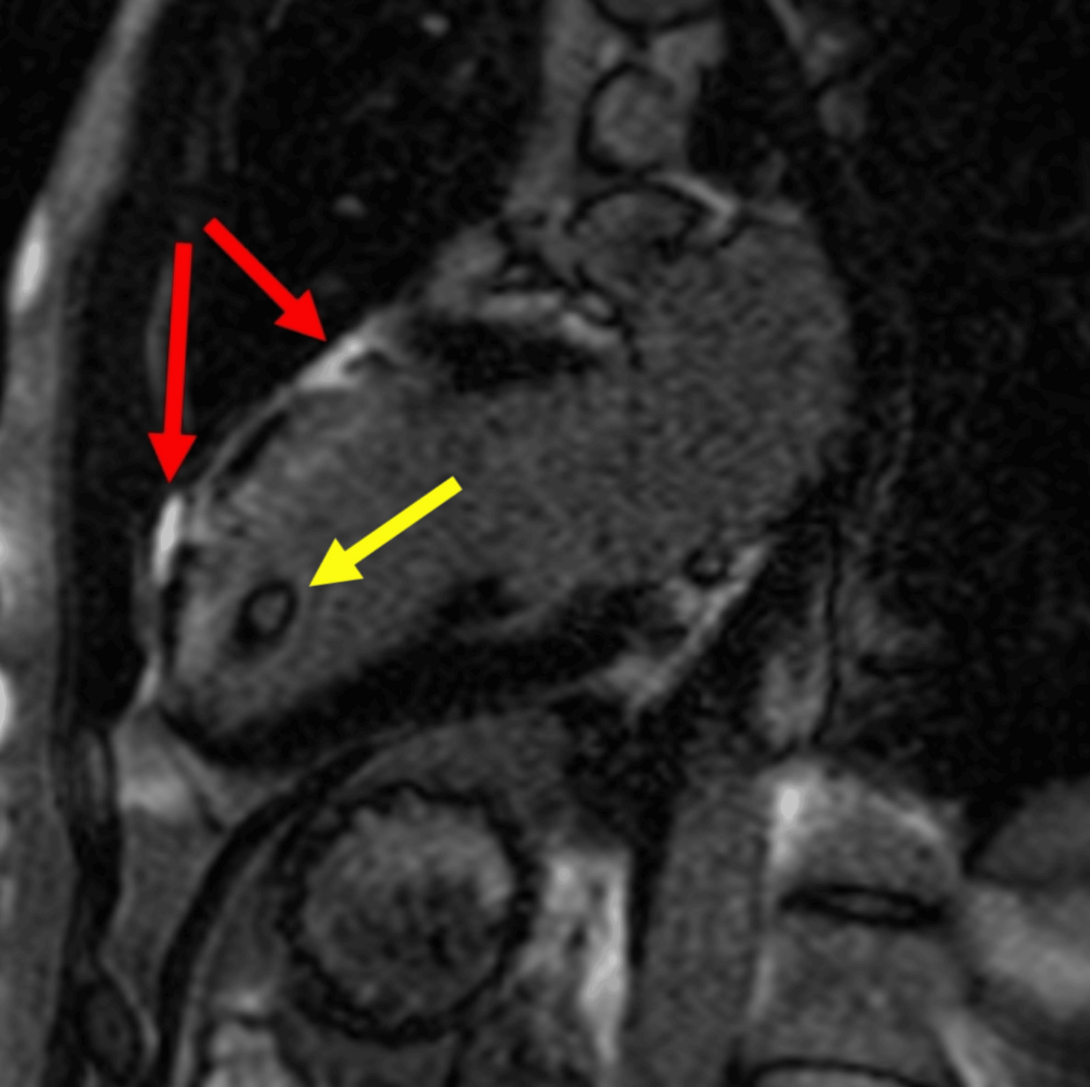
Cardiac magnetic resonance imaging scan (two-chamber view) demonstrating late gadolinium enhancement in the anterior segment of the heart, indicating left anterior descending artery territory infarction (highlighted by red arrows). A thrombus is also visible at the left ventricular apex (highlighted by yellow arrow)
